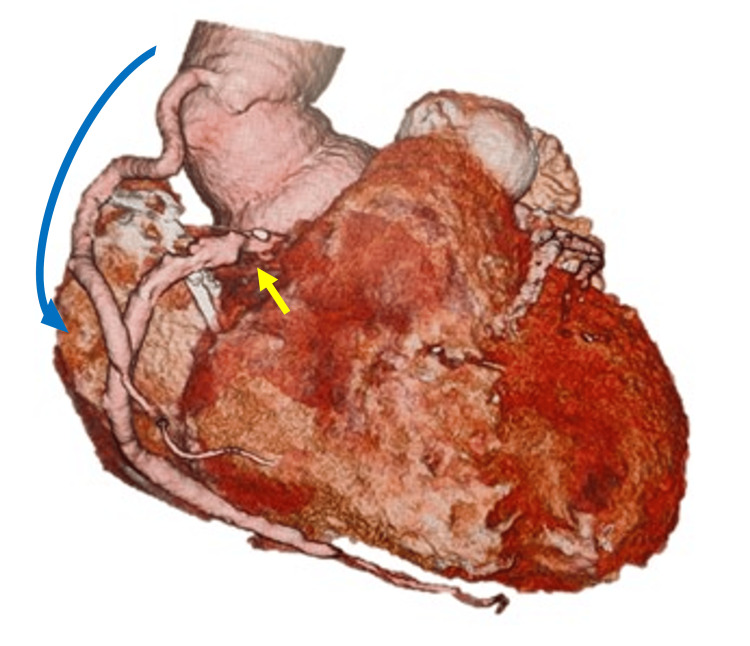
Coronary computed tomographic angiography after surgery Coronary artery bypass grafting from the vascular graft to the right coronary artery was performed (blue arrow). The proximal portion of the right coronary artery was ligated (yellow arrow).
